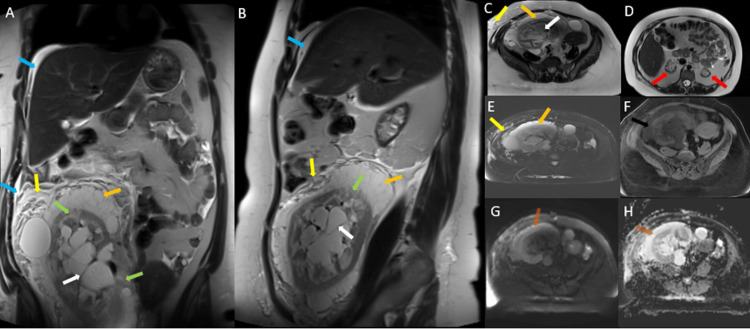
MRI of the abdomen: (A) coronal T2W; (B) sagittal T2W; (C & D) axial T2W; (E) T2W fat saturation (fat sat); (F) T1W fat sat; (G) DWI and (H) ADC images: showing an enlarged transplanted kidney in the right iliac fossa with linear cortical and peri-pyramidal high T2 signal (green arrows); septated peri pelvicalyceal cystic structures (white arrows); septated perinephric fluid (orange arrows) which appear hypointense on T1W fat sat (black arrow), with traces of fluid in the abdominal wall (yellow arrows); mild free fluid in the upper abdomen and pelvis (blue arrows) and no diffusion restriction (brown arrows). Native kidneys are atrophic (red arrows). T2W: T2-weighted image; T1W: T1-weighted image; DWI: diffusion weighted imaging; ADC: apparent diffusion coefficient
